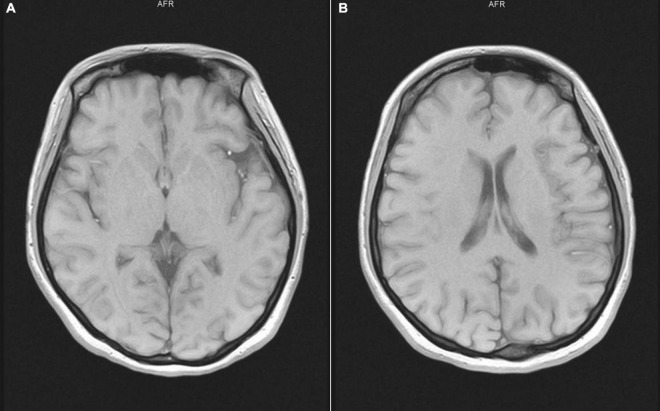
Cranial magnetic resonance scan showed microischemia on the bilateral frontal lobe. (A,B) show that different sections of the patient’s head MRI are normal.
